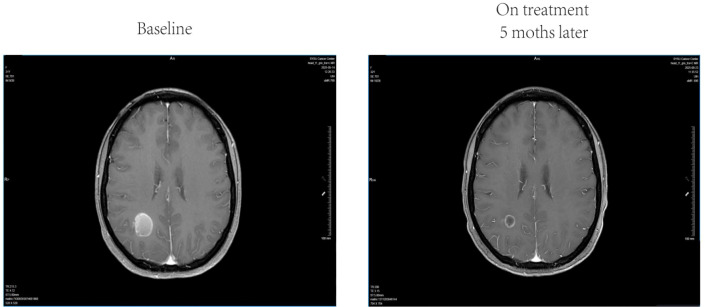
Imaging case of intracranial partial response. A 32-year-old female with melanoma brain metastases treated with a hypofractionated radiotherapy regimen of 6 Gy × 6 fractions. The magnetic resonance imaging (MRI) shows partial response at 5-month follow-up.
